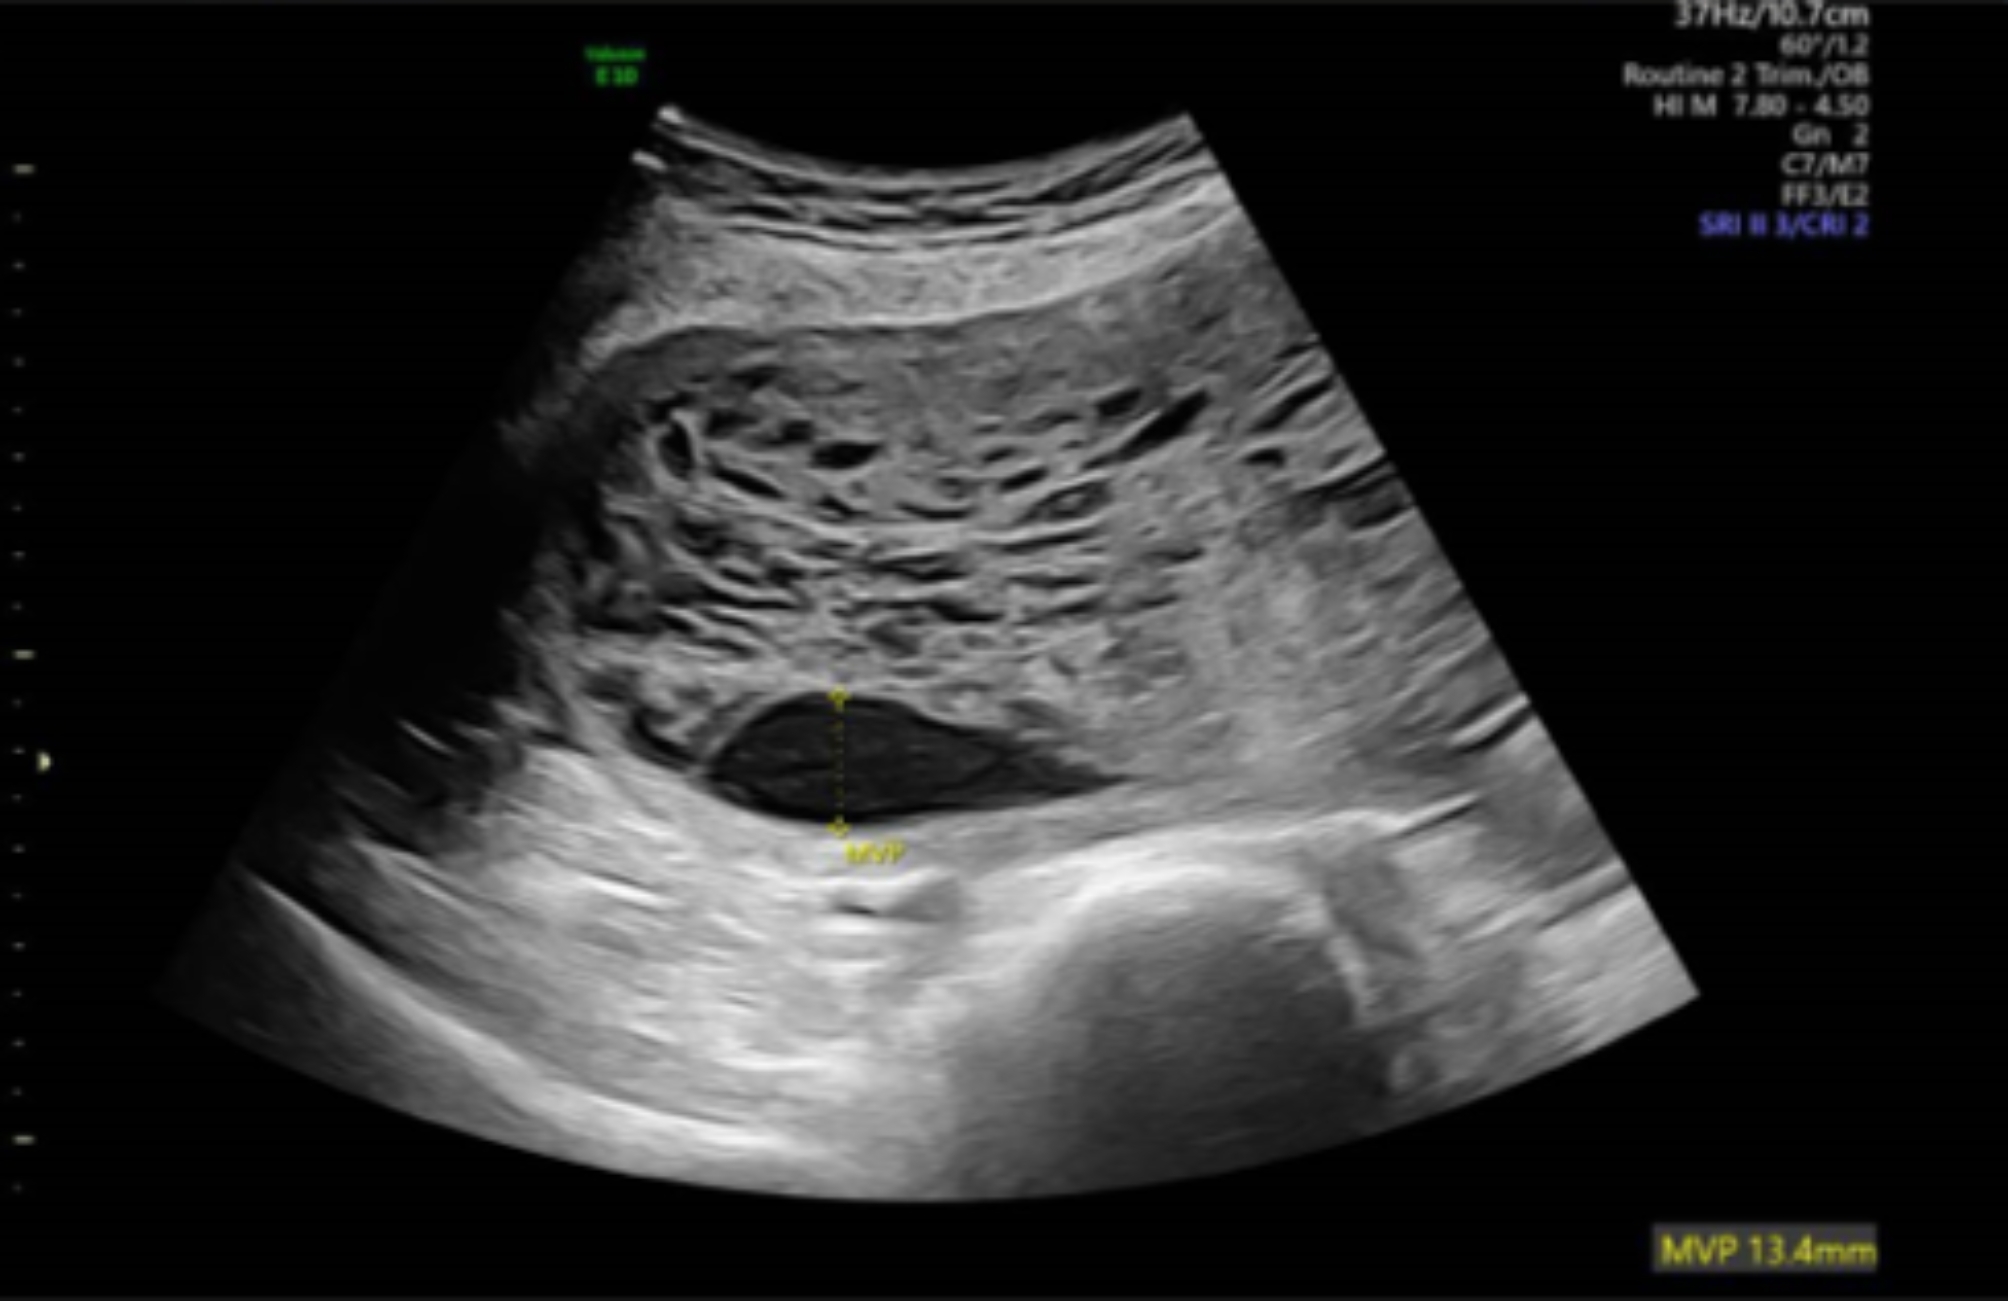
Ultrasound scan at 16 weeks showing an enlarged multicystic placenta and oligohydramnios
